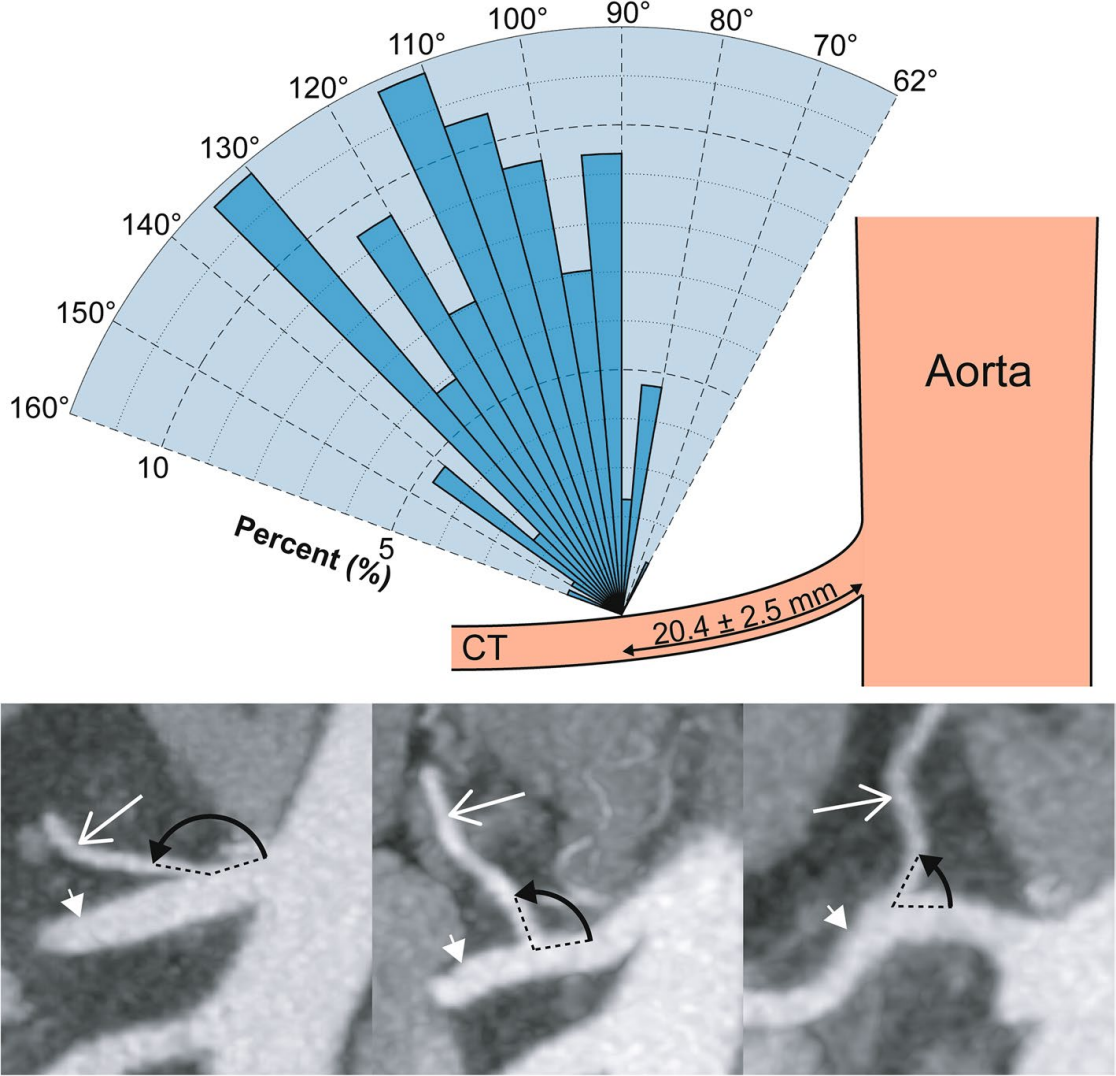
Angle of the origin of the LGA from the coeliac/coeliacomesenteric/gastrosplenic trunk in the para-sagittal plane. Histogram of the angle of origin of LGA from the parent vessel plotted as a percentage of participants. The minimum and maximum angle is represented by the radial range of the graph (62 to 160°). Inset CT images show examples and calculations for the LGA clockwise angle from participants in the (left to right) highest, median and lowest decile. Long white arrow, LGA; short white arrow, coeliac trunk; black arrow, measured angle
